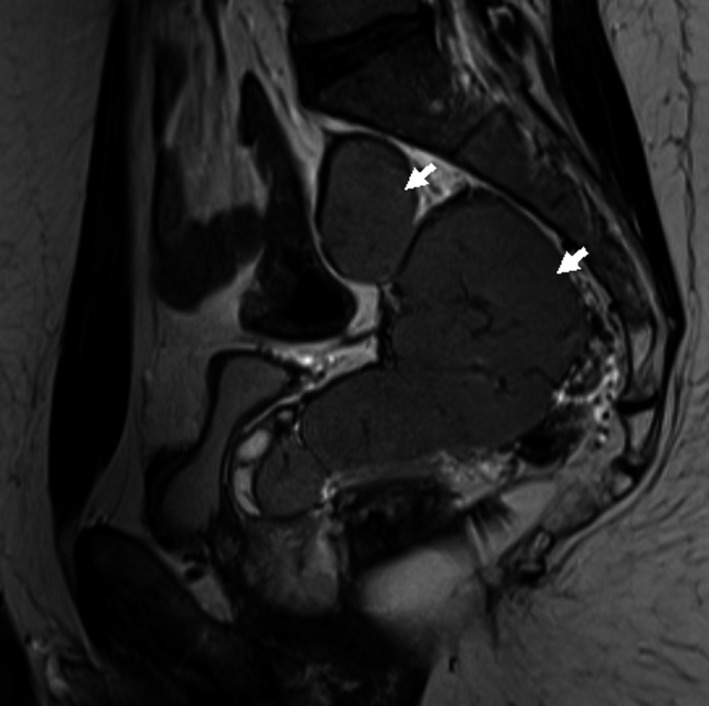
Sagittal view of a magnetic resonance imaging (MRI) of the pelvis, T2‐weighted sequence, showing the same huge pelvis mass consisting of two adjacent well‐defined masses in the right perirectal space, the larger measuring 10 × 8 cm, compressing the right seminal vesicle and rectum
